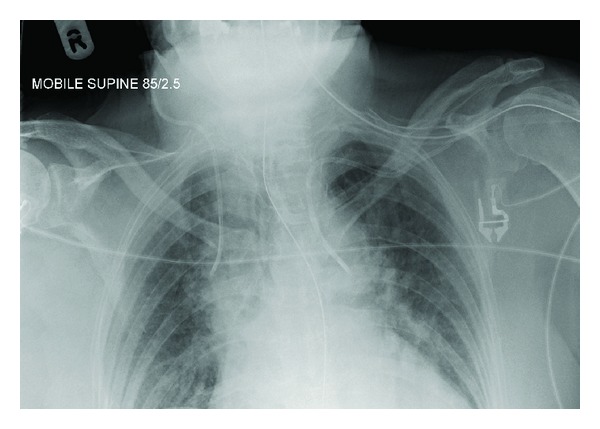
Chest radiograph showing abnormal position of the left subclavian line.
